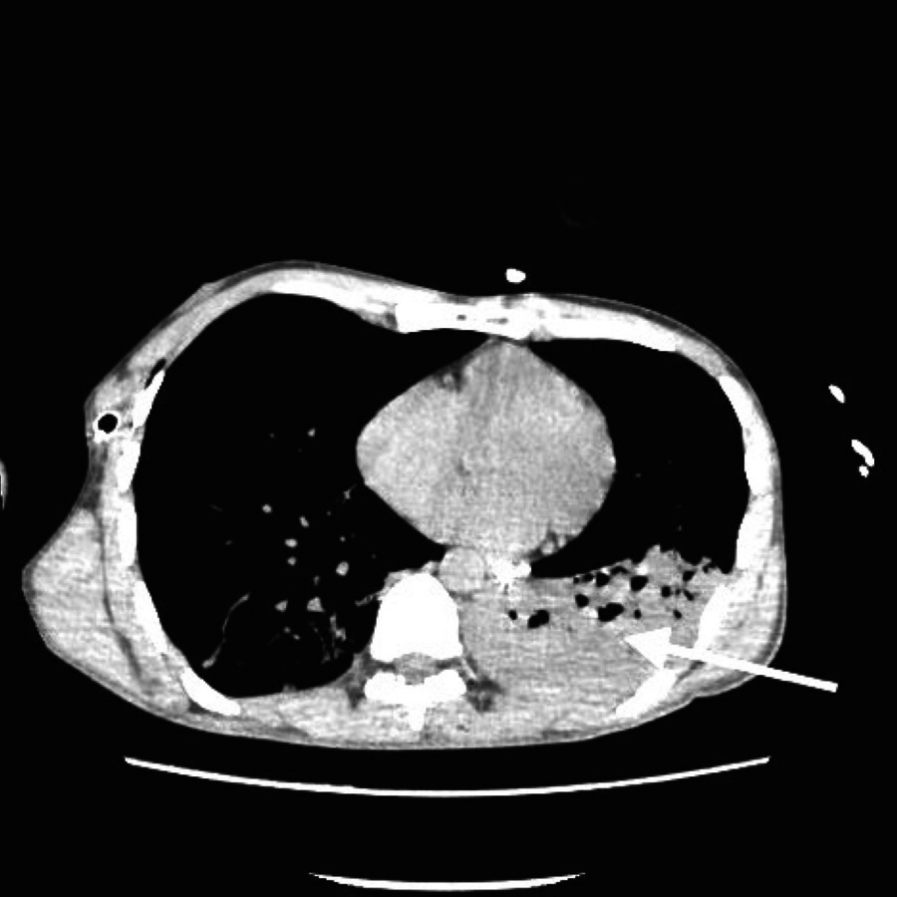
Computed tomography showing large left lower lobe consolidation.
